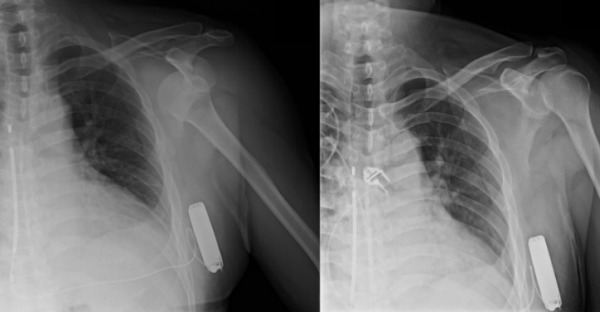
Radiographs showing (left panel) anterior glenohumeral dislocation and (right panel) return of the humeral head to the glenoid cavity after closed reduction. Also seen is the S-ICD system.
